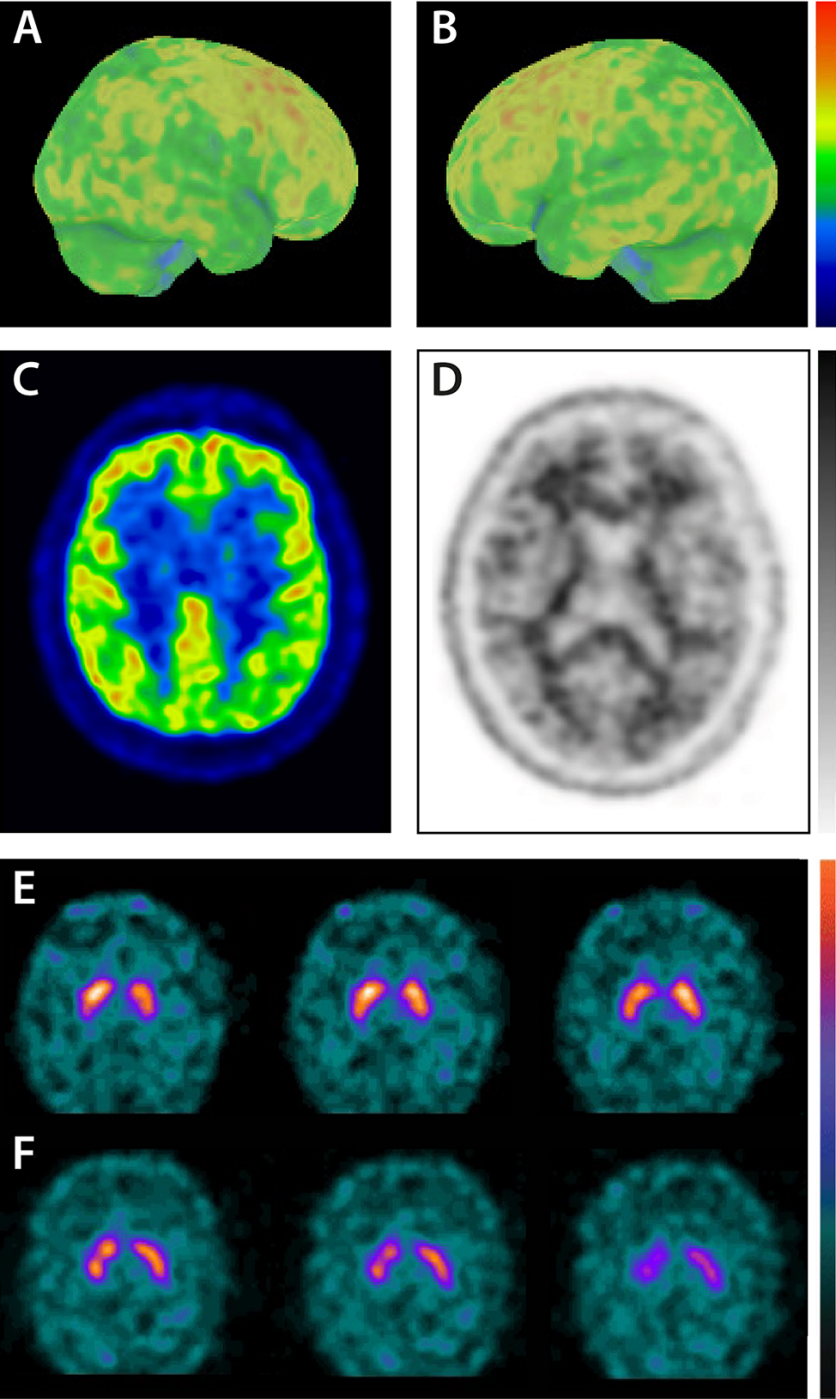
Imaging biomarkers. (A–C) 18F-FDG-PET results. Three-dimensional surface projections (A, B) show distinct bilateral temporo-parietal and occipital hypometabolism. (A) Right hemisphere; lateral view. (B) Left hemisphere; lateral view. (C) Hypometabolism is relatively spared in the posterior cingulate gyrus compared to occipital areas (cingulate island sign); transverse view. (D) 18F-Florbetaben-PET did not show increased amyloid burden. (E, F) Dopamine transporter imaging with 123I-FP-CIT SPECT in transverse slices. While the initial scan was negative (E), follow up showed lower uptake within the right putamen (F).
